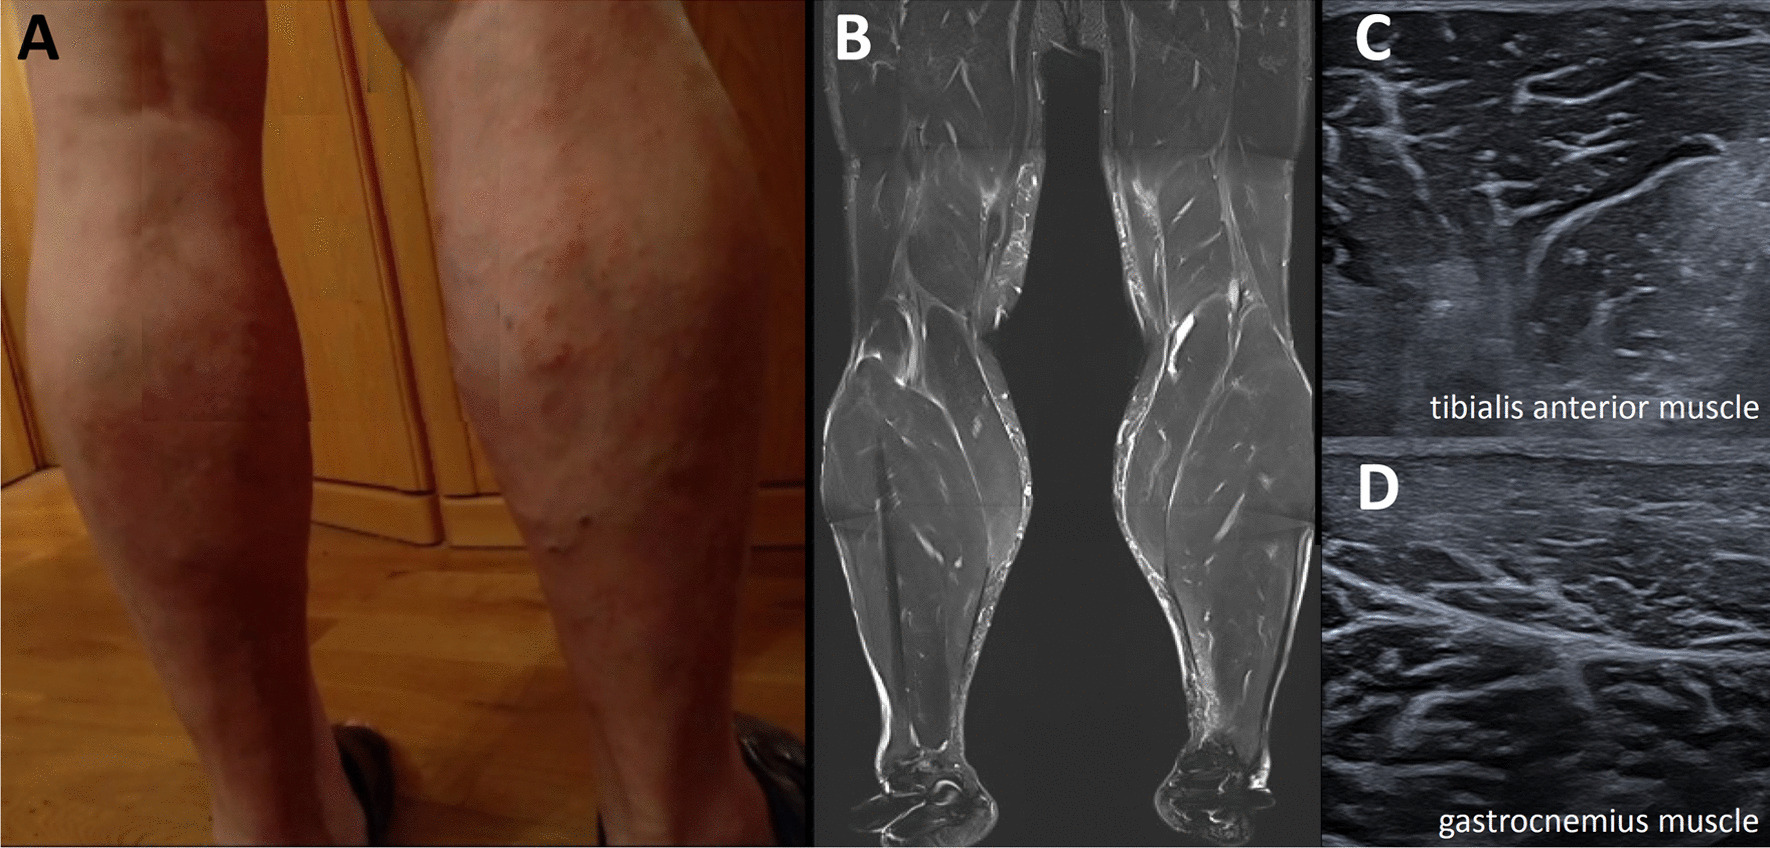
Calf muscles of the patient. A Photograph. B Muscle MRI. C and D Muscle ultrasound
